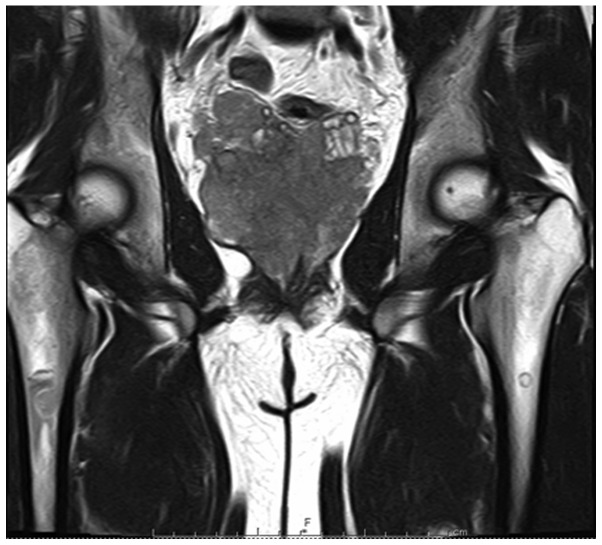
Coronal T2-weighted magnetic resonance image demonstrating a large tumor in the prostatic legion.
